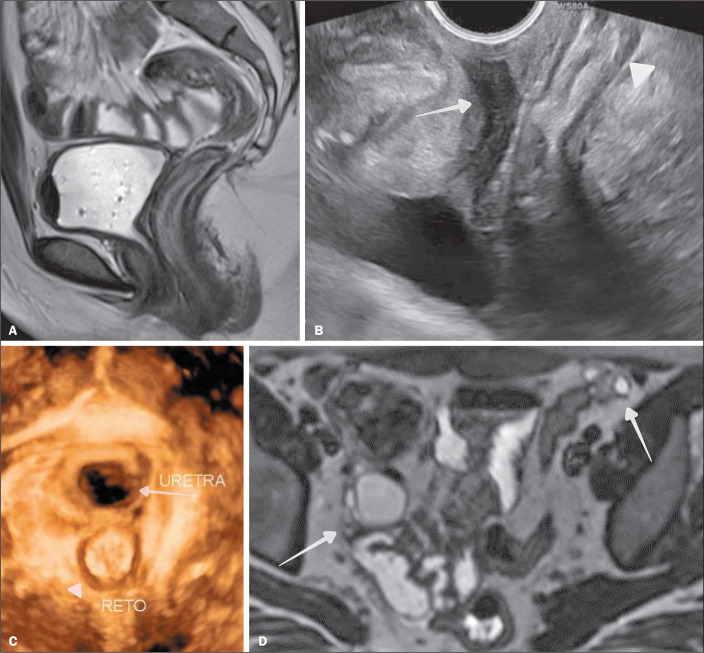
Mayer-Rokitansky-Küster-Hauser syndrome. Sagittal T2-weighted
